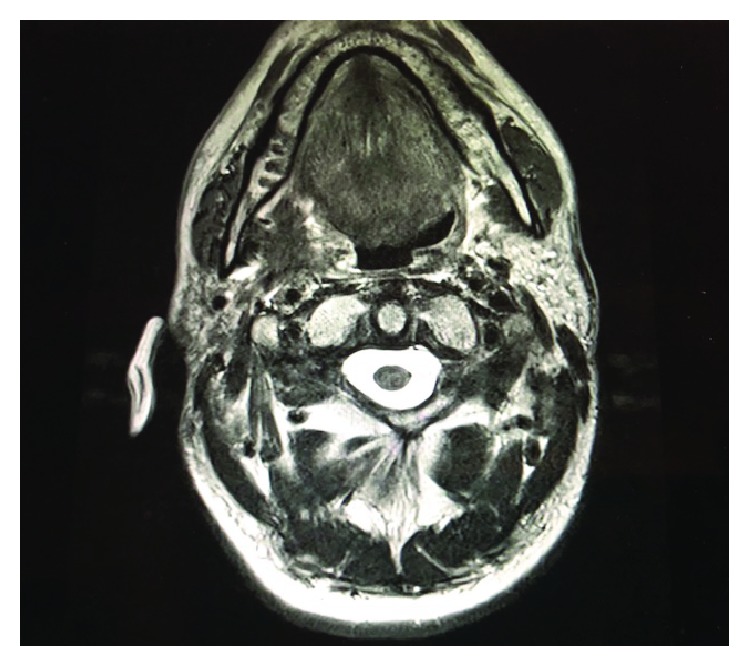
MR image of the solid tumor before pembrolizumab treatment.
